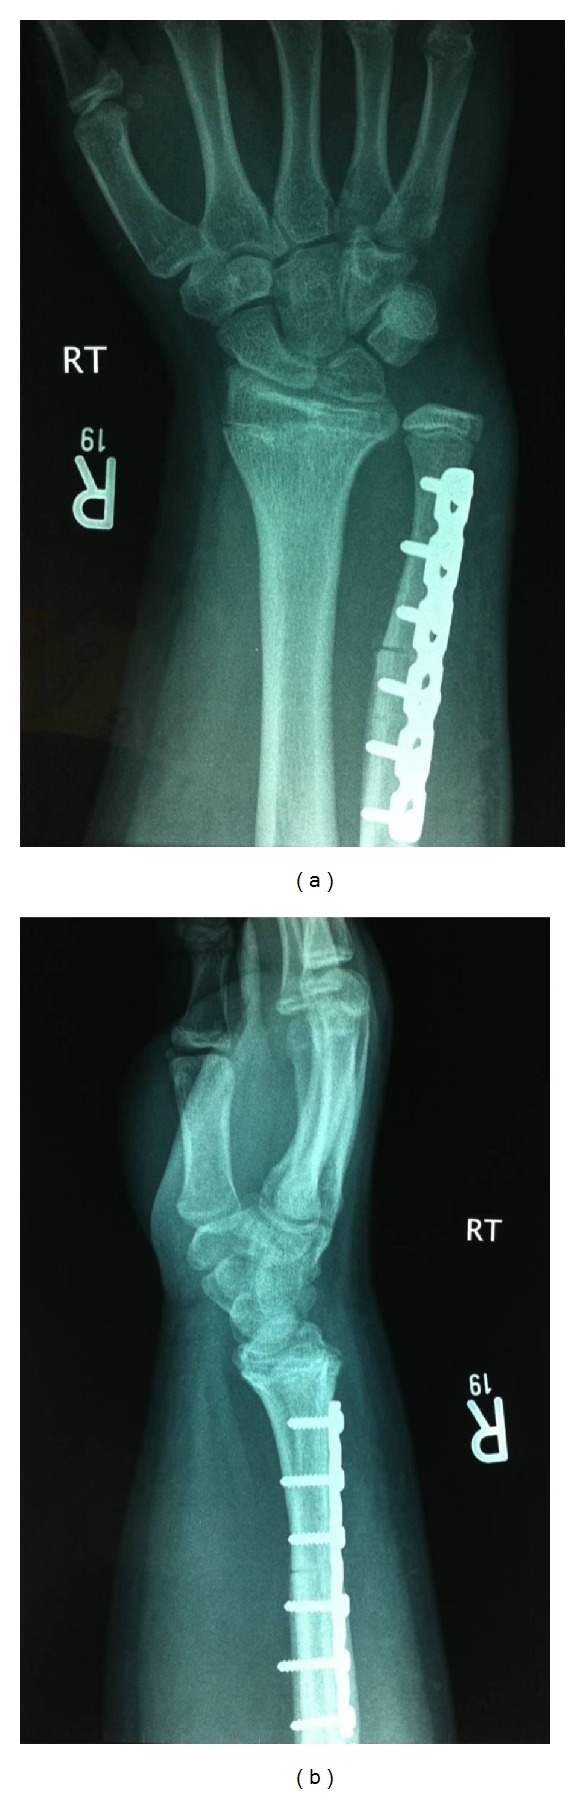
Postoperative anteroposterior and lateral radiographs demonstrating satisfactory ulnar shortening and fixation of the osteotomy site.
